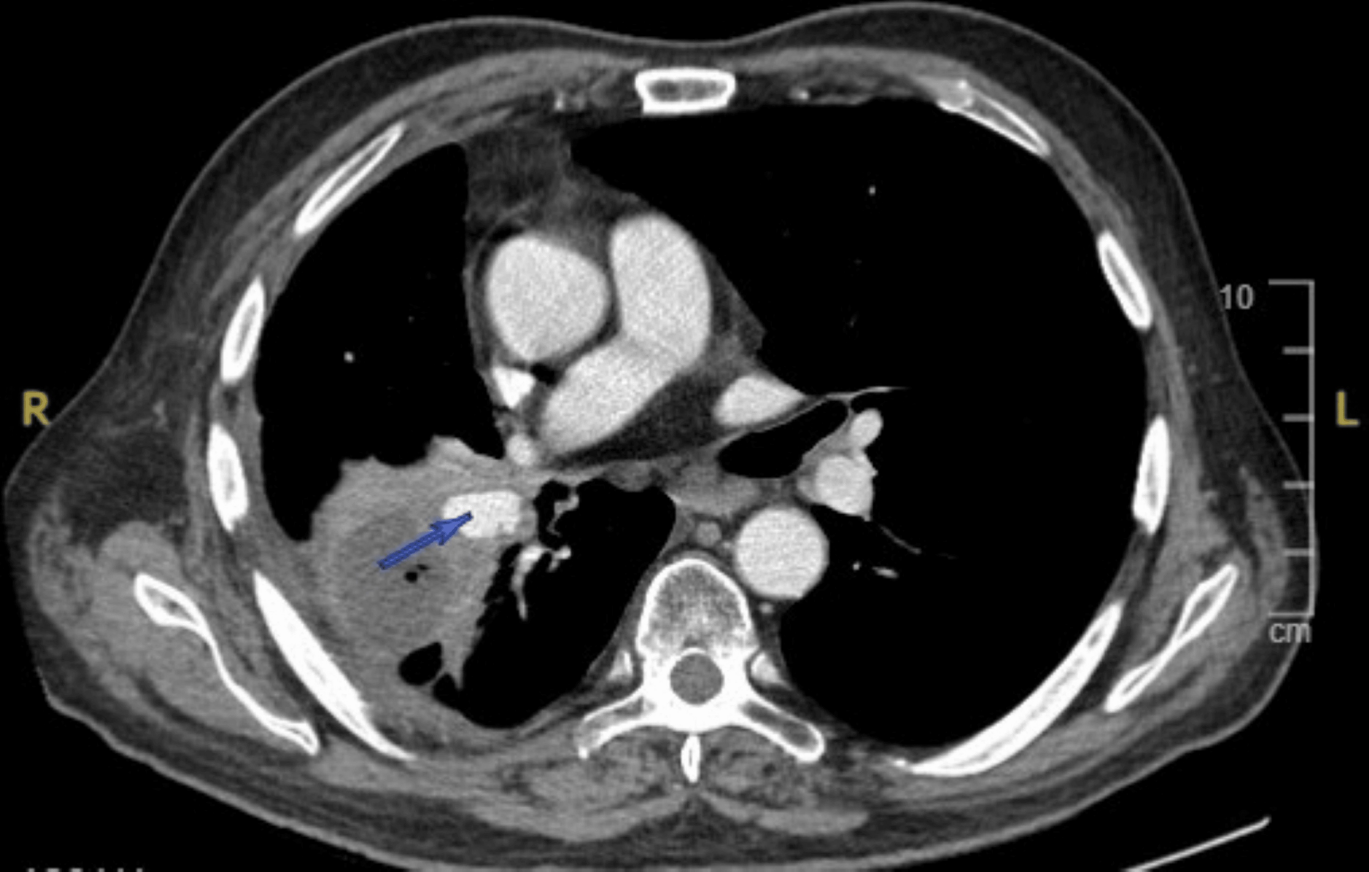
Computed tomography (CT) scan with intravenous (IV) contrast of the chest showing a pulmonary artery pseudoaneurysm (arrow) arising from the right inferior pulmonary artery, adjacent to a necrotic lung tumor.
